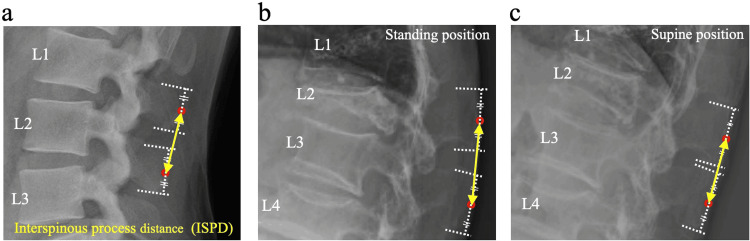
Radiographic measurement of interspinous process distance (ISPD). (a) Definition of ISPD as the linear distance between the midpoints of adjacent spinous processes on lateral radiographs. (b) Standing position and (c) supine position radiographs of a representative case with an L2 osteoporotic vertebral fracture, used for ΔISPD calculation. Digitally drawn open circles indicate the midpoint of each spinous process used for ISPD measurement in panels (b) and (c). Measurements were performed on 150% magnified images to improve reliability as previously described for cervical ISPD. ΔISPD was defined as standing ISPD minus supine ISPD; normalized ΔISPD was ΔISPD divided by L4 anterior vertebral height multiplied by 100.
